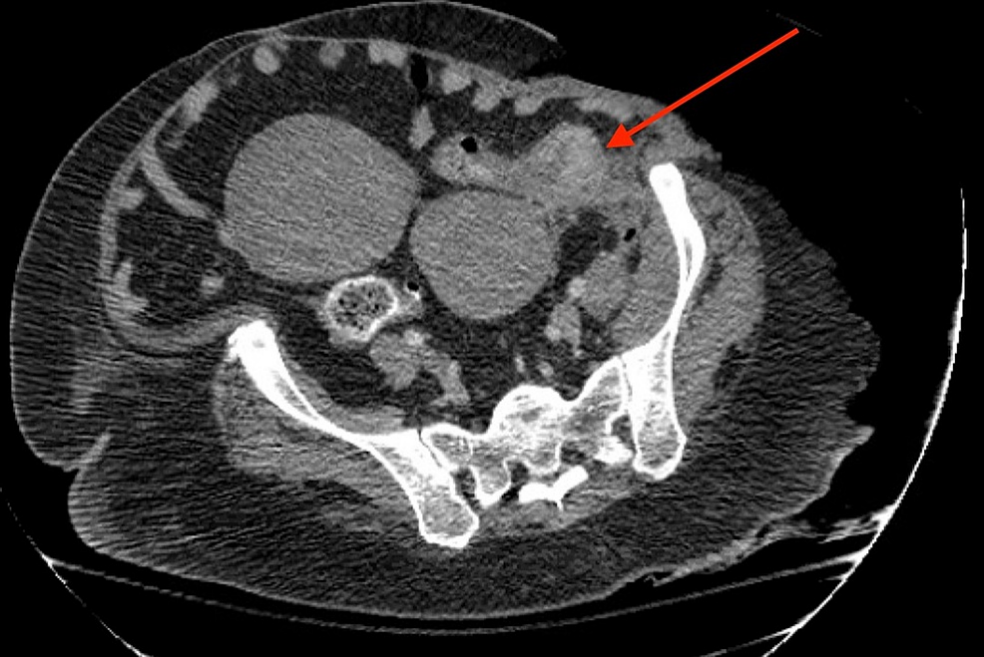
CT abdomen and pelvis showing thickening of sigmoid colon
